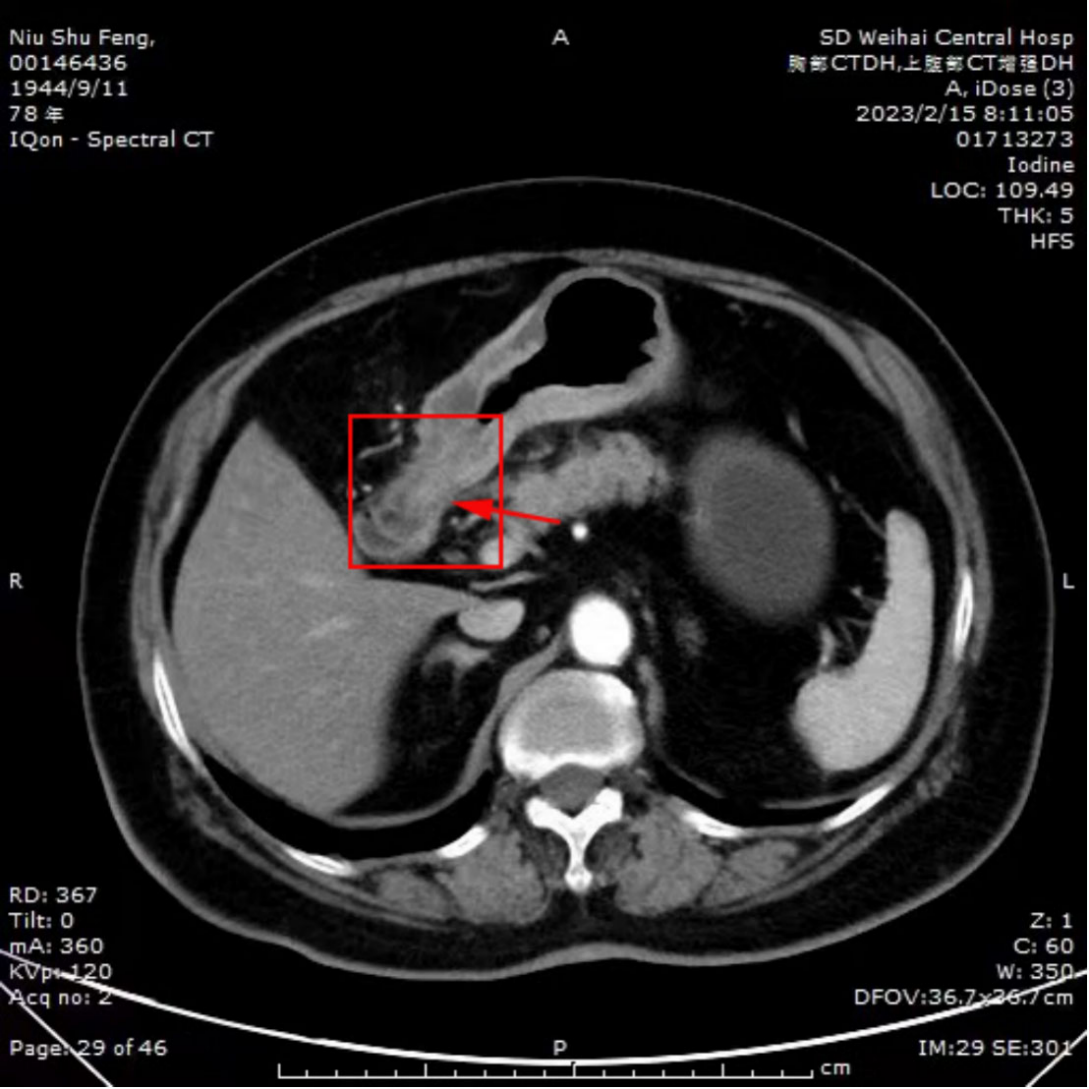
CT shows diffuse abnormal enhancement of gastric body and sinus wall.
